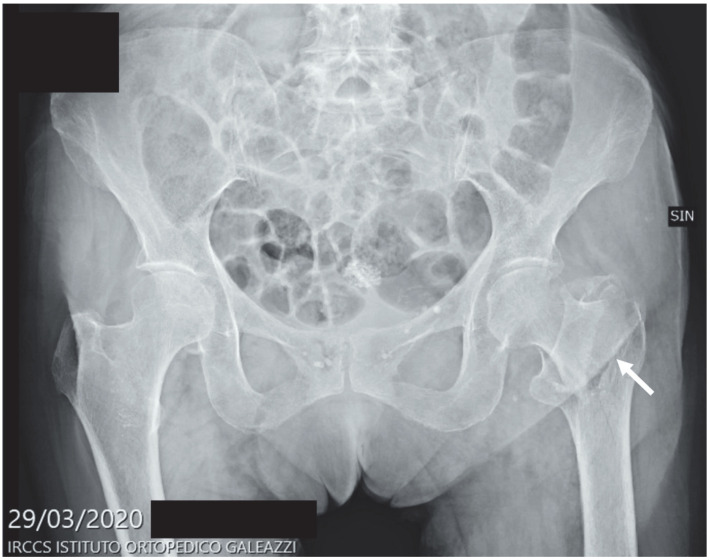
Figure shows a frontal pelvis X-ray examination of an 87-year-old female patient who suffered a left pertrochanteric femoral fracture (arrow) that has been surgically treated with an intramedullary nail.
